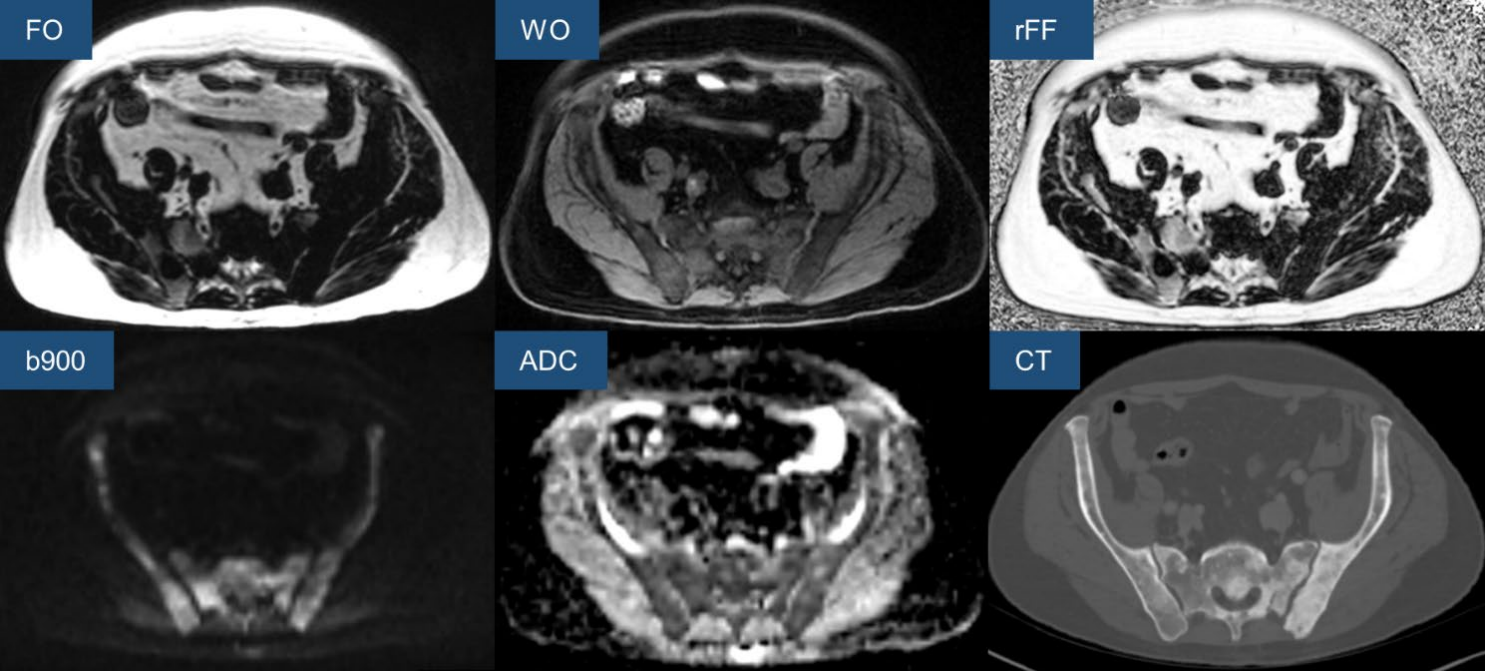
Multiparametric bone MRI imaging protocol for CT-guided bone biopsy target lesion selection including DWI and T1-weighted, gradient-echo Dixon images, CT of the same area for comparison, FO – fat-only Dixon image, WO – water-only Dixon image, rFF – relative fat fraction, b900 – high b-value DWI with corresponding ADC map
